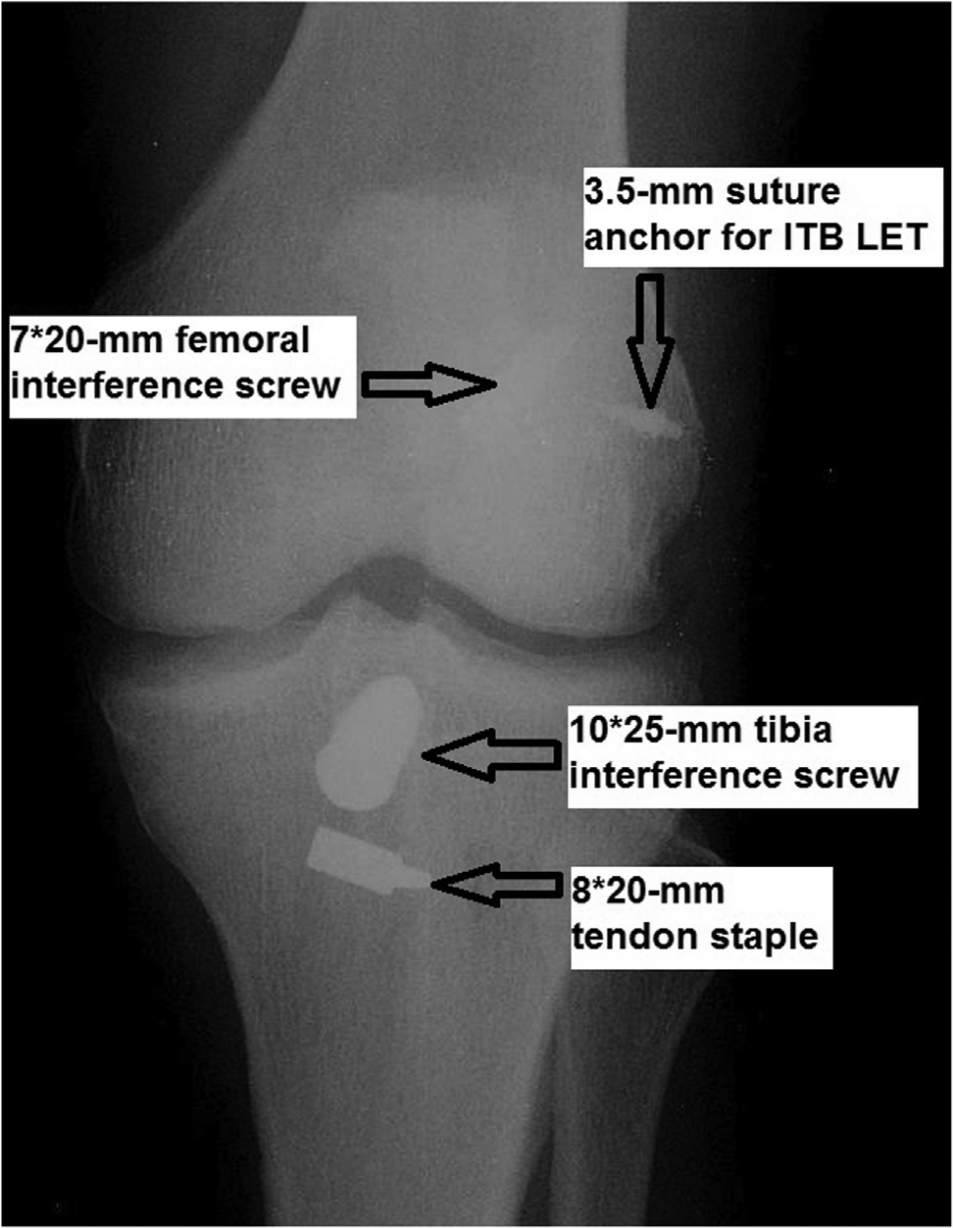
Postoperative anteroposterior radiograph of the left knee showing hardware locations and confirming patella integrity.
